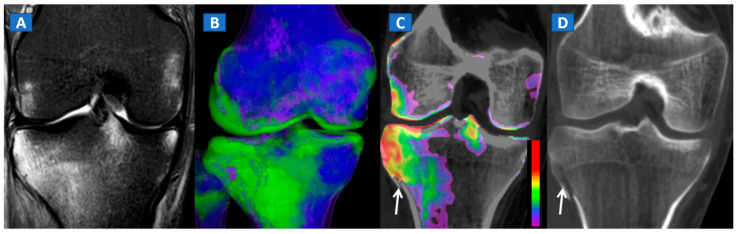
A 34-year-old male with a post-traumatic avulsion fracture of the lateral aspect of the proximal tibia. On the coronal STIR MRI image (A), multiple BME foci can be identified on the lateral aspect of the proximal tibia. On the coronal 3D DECT image (B), the distribution of BME foci is confirmed. On the corresponding 2D 1 mm reconstructed 2D DECT image (C), the BME appears more pronounced on the lateral aspect of the proximal tibia, adjacent to a cortical defect (arrow). The corresponding standard 1 mm reconstructed coronal CT image (D) confirms a minimally dislocated avulsion fracture (arrow).
